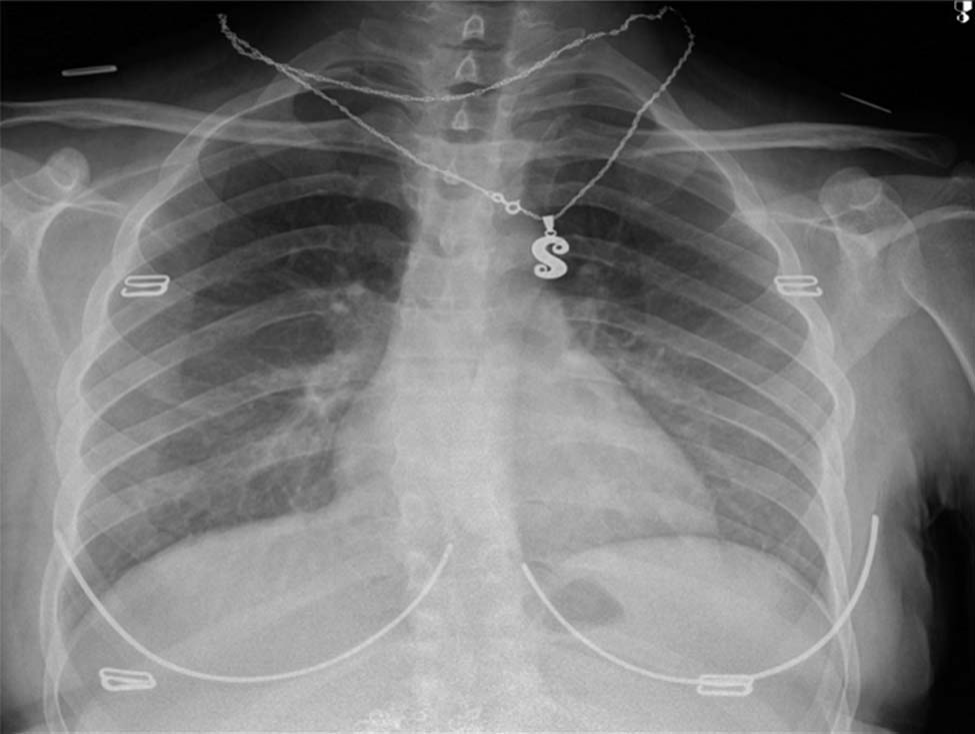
Normal erect posteroanterior (PA) chest X-ray.
